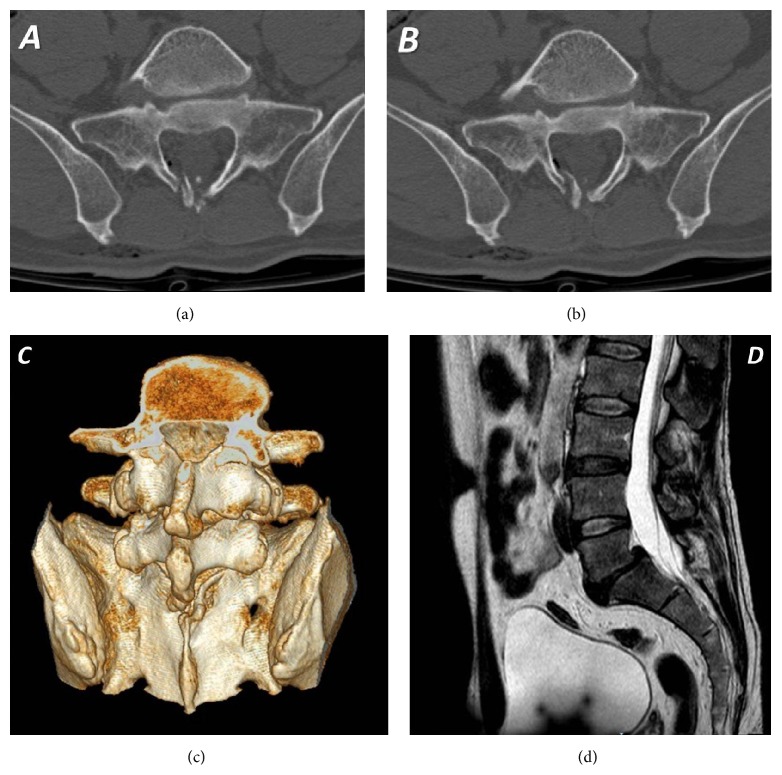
Lumbosacral CT scan ((a), (b), (c)) and RMI (d) showing a multifragmented fracture of the S1 lamina, with air bubbles in the epidural space.
